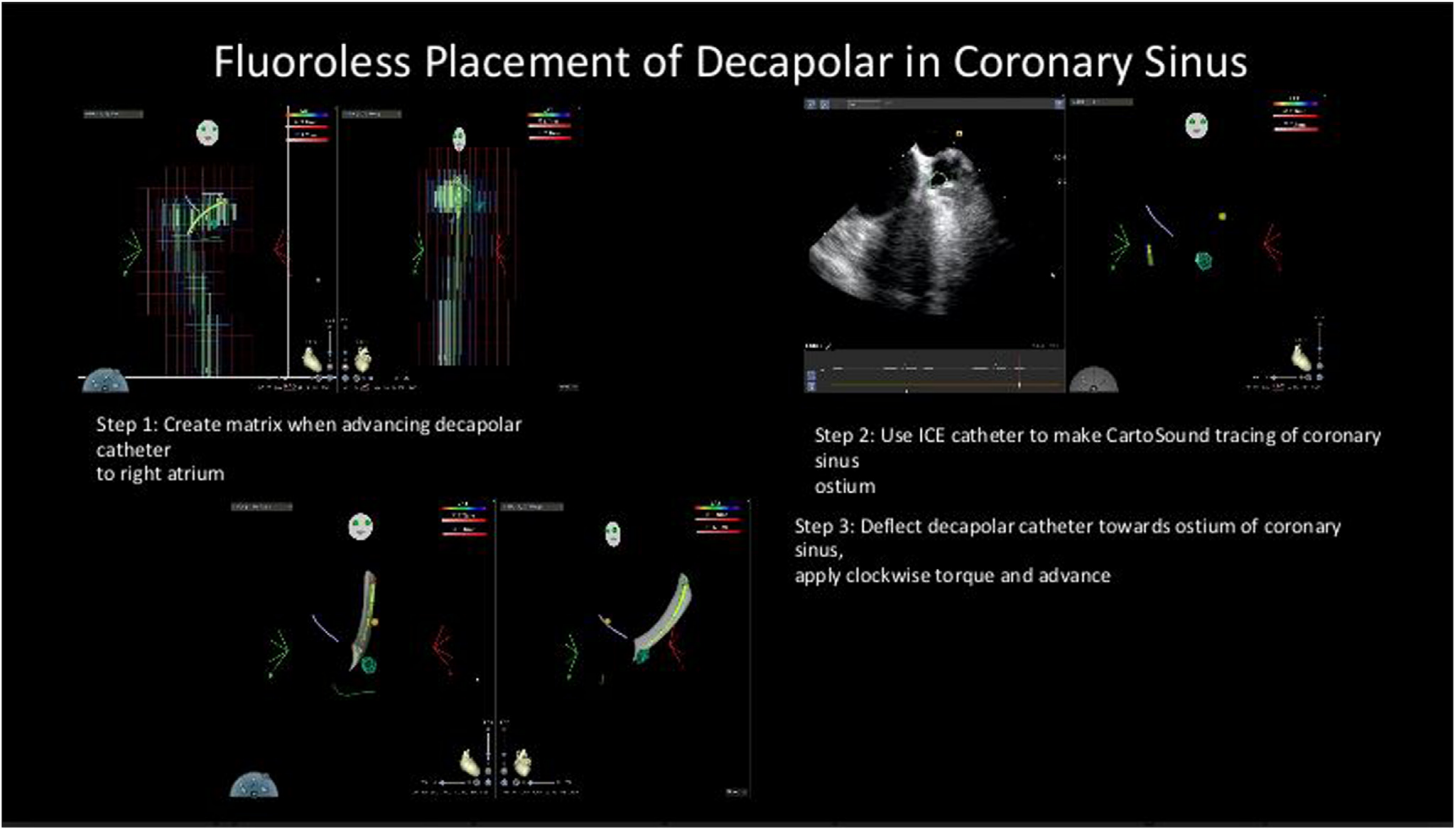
Zero-fluoroscopy DECANAV catheter (Biosense Webster) placement in coronary sinus (CS) (representative images obtained from a recent case). Step 1: Advancement of DECANAV catheter by feel, with simultaneous matrix and fast anatomic map creation and marking of His bundle. Step 2: CARTOSOUND mark-up of CS os. The right atrium / CS os are on the right side of screen and left atrium / mitral annulus are on the left side of screen on the intracardiac echocardiography (ICE) images. Step 3: Deflection of DECANAV catheter from His location to CS os and clockwise torque to advance into the CS.
